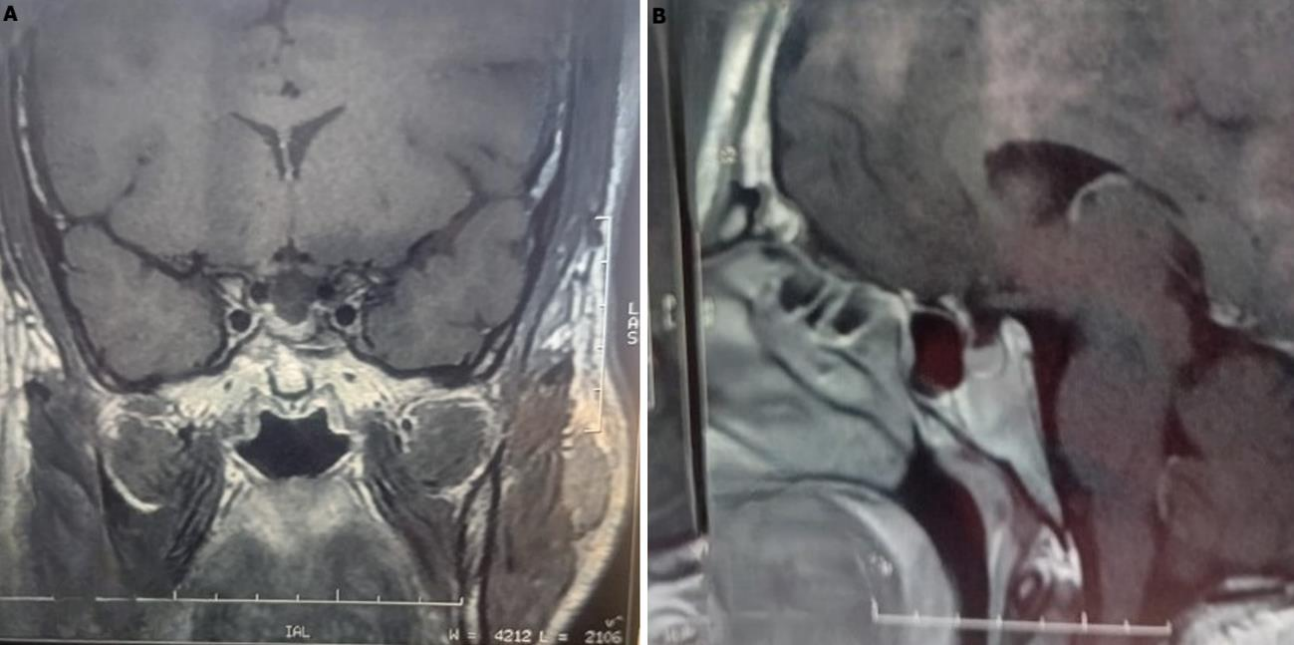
T1-weighted MRI of the hypothalamic-pituitary region (A) coronal; B) suprasellar arachnoid cyst with downward herniation of the suprasellar cistern; the posterior pituitary bright spot is absent, while the anterior pituitary gland is preserved
